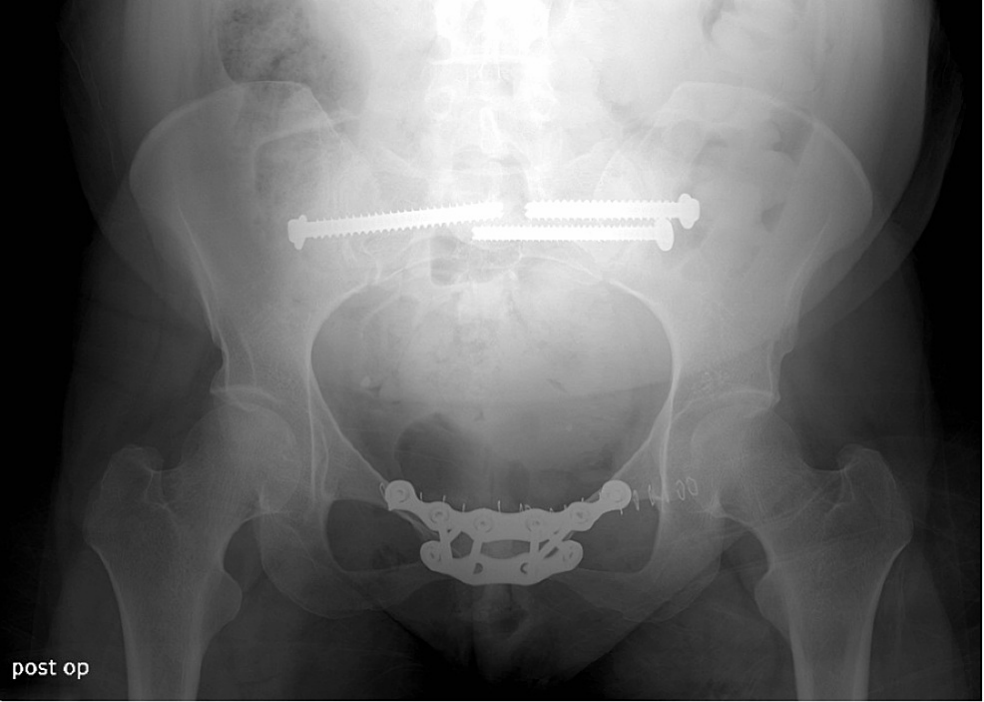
Anteroposterior (AP) radiographic view of the patient's pelvis taken postoperatively, demonstrating the orthopedic hardware in position
